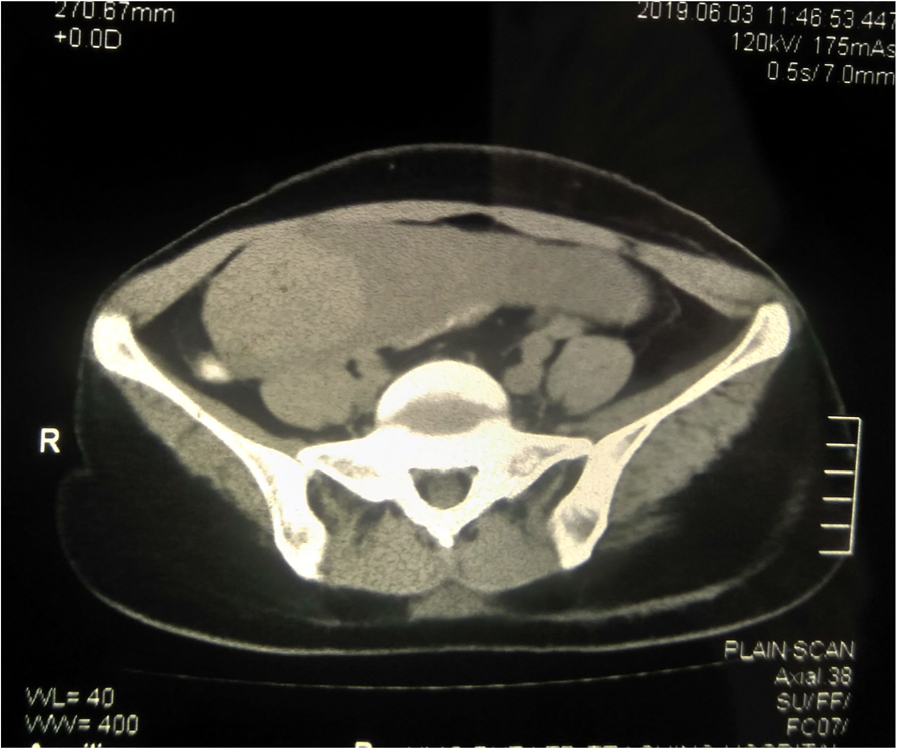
Computed tomography scan of pelvis (axial view) showing solid cystic lesion of left ovary extending to right pelvic region
